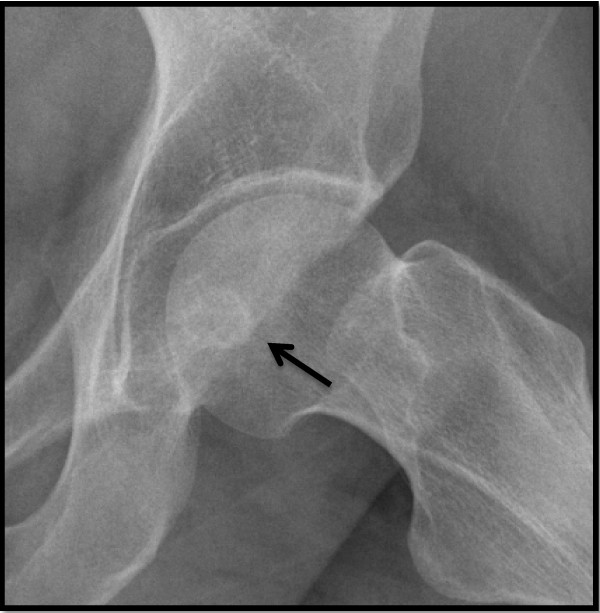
Plain radiograph frog leg lateral views (black arrow) showing resolution of the lesion.
